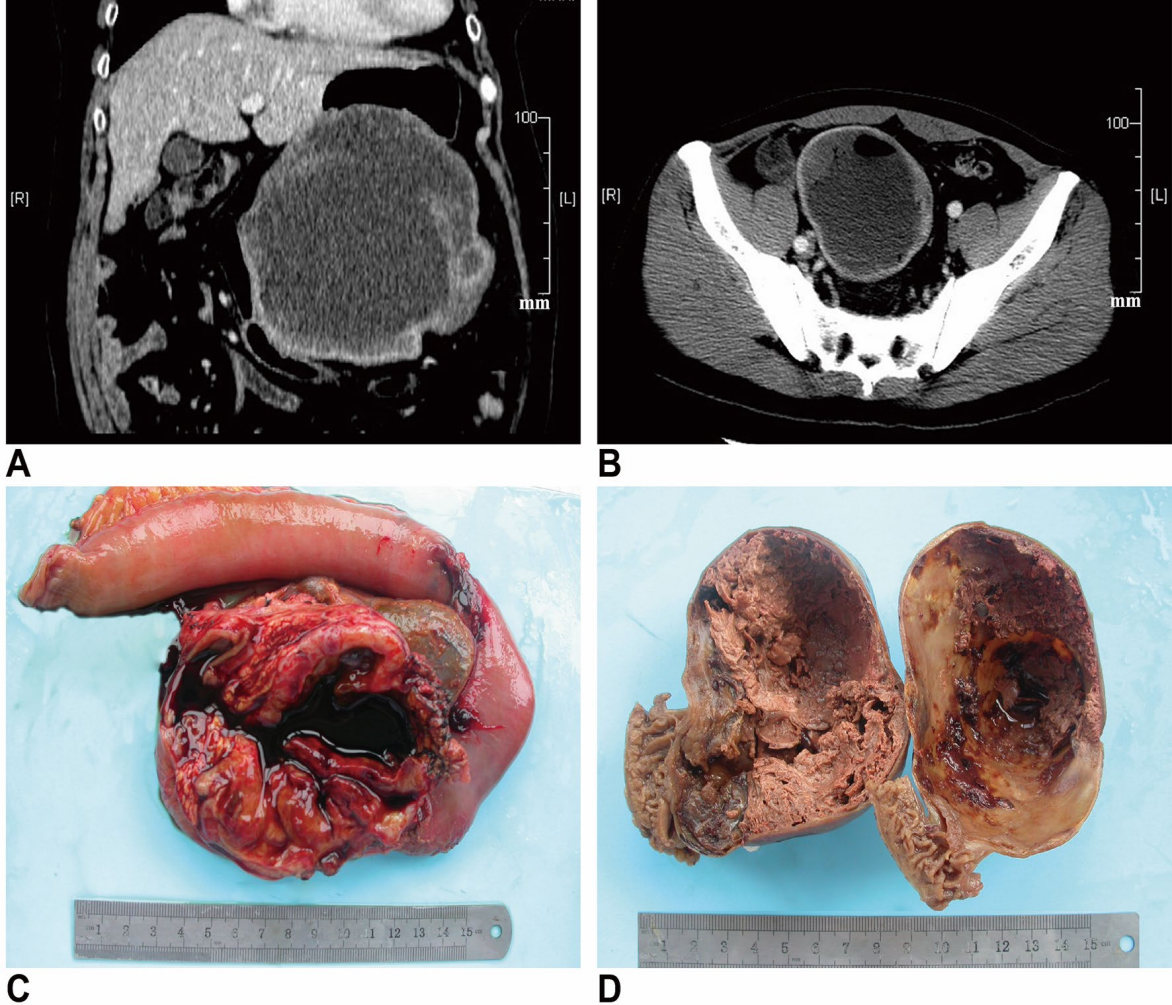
On contrast-computed tomography (CT), cGISTs usually demonstrate an exophytic, well-defined, low-density mass with peripheral enhancement (a, b). Grossly, the cystic component made up the vast majority of the masses and was surrounded by neoplastic parenchyma variable in thickness. Most of the cysts were filled by dark bloody serous fluid and necrotic debris (c, d)
